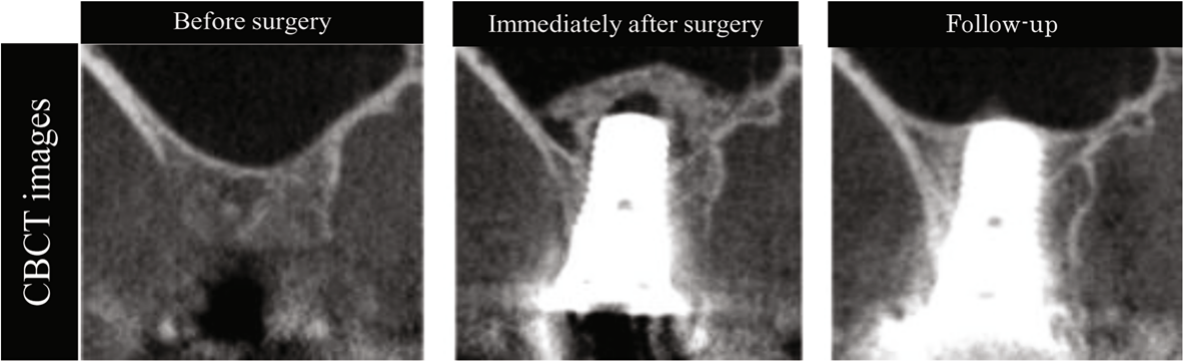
Treatment protocol for the present study. CBCT was performed before surgery, immediately after surgery, and at follow-up (≥ 3 years). CBCT, cone-beam computed tomography
